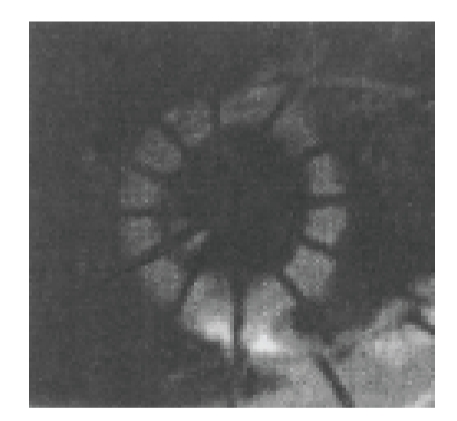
Radially tagged cardiac MRI taken from [26].
